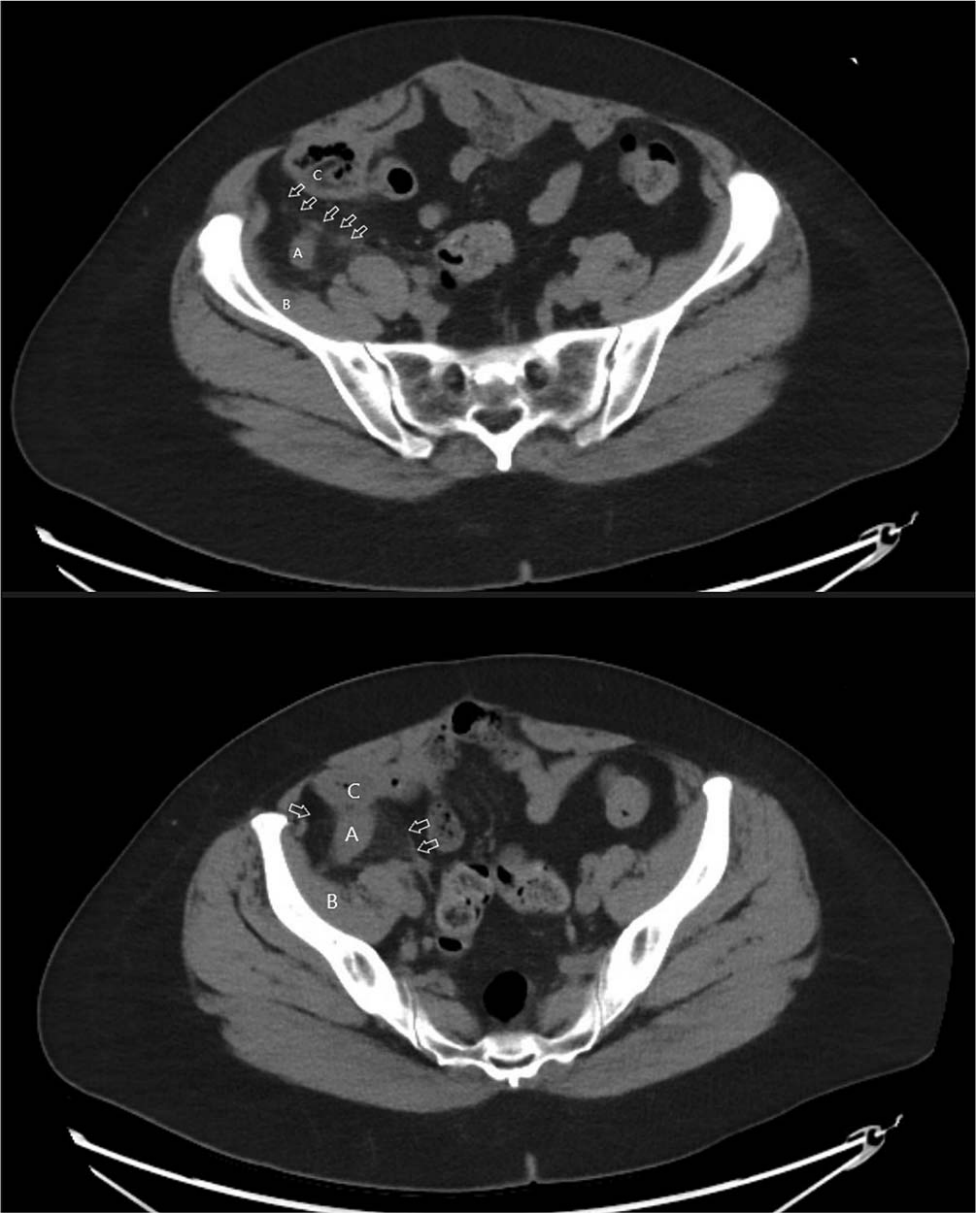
Axial images of CT scan with IV contrast showing appendix within retrocecal position in proximity within iliacus space. (A) Appendix; (B) iliacus muscle; (C) cecum.
